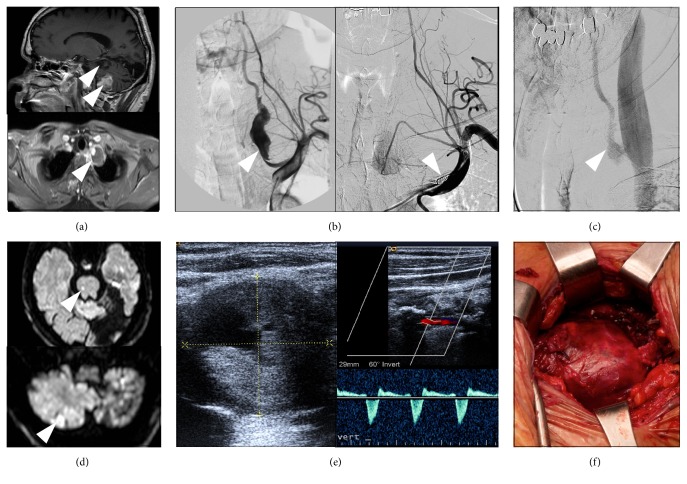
(a) (Top) sagittal T1-weighted contrast enhanced brain magnetic resonance imaging (MRI) scan showing a subacute ischemic lesion of the inferior left cerebellar hemisphere in the left posterior-inferior cerebellar artery (PICA) territory and chronic cerebellar and occipital ischemic lesions; (bottom) axial T1-weighted contrast enhanced MRI image of the neck showing a giant fusiform aneurysm (arrow), containing an eccentric thrombotic formation, originating from the left vertebral artery. (b) Left subclavian angiograms showing (left) the displasic and tortuous aspect of the giant aneurysm and (right) aneurysm exclusion after the endovascular treatment by deposition of GDC vortex spirals in the proximal segment of left vertebral artery (VA) (arrow). (c) Late sequences of right vertebral angiogram performed six years after the endovascular procedure showing full revascularization of the rostral part of the aneurysm by retrograde blood flow from the patent vertebrobasilar axis. (d) Diffusion-weighted imaging (DWI) brain MRI showing new acute ischemic lesions of the cerebellum and the brainstem (arrows). (e) Sonography and Color Doppler Ultrasound of neck vessels showing the aneurysm (3.5 cm) and the biphasic flow in the left vertebral artery supportive of the embolic etiology of the new ischemic stroke. (f) Surgery of the giant aneurism by aneurysmorraphy with thrombectomy: aneurysm exposure and isolation from the surrounding tissue.
